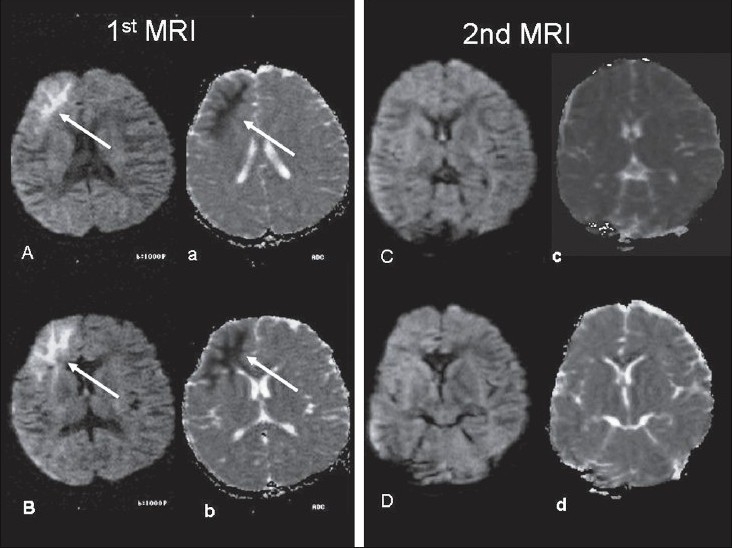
First MRI (A, a; B, b): DWI (A, B) images show hyperintensity (white arrow) involving the grey and white matter in the right basifrontal region with ADC images (a, b) showing hypointensity (white arrow) in the same region, suggesting cytotoxic edema and restricted diffusion. Repeat MRI after 4 days with DWI (C, D) and ADC (c, d) images, shows resolution of the restricted diffusion
